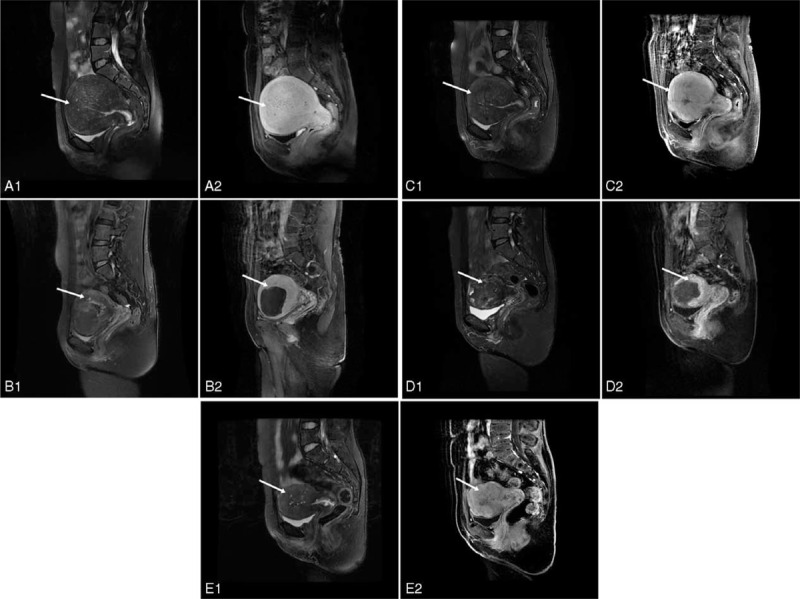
A 44-year-old woman who underwent twice USgHIFU ablation for symptomatic adenomyosis. (A1-A2) Sagittal view of MR image obtained before the first USgHIFU ablation showed diffuse junctional zone thickening of the uterus (arrows). The volume of the uterus was 581 cm^3^. (B1–B2) Sagittal view of MR image obtained 3 months after first USgHIFU ablation showed decreased size of the uterus. The volume of the uterus was 371 cm^3^. Arrows indicate nonperfusion area (183 cm^3^) after USgHIFU. (C1–C2) Sagittal view of MR image obtained 48 months after first USgHIFU ablation (before second USgHIFU ablation) showed enhancement of the treated area (arrows). The volume of the uterus was 319 cm^3^. (D1–D2) Sagittal view of MR image obtained 12 months after second USgHIFU ablation showed decreased size of the uterus. The volume of the uterus was 150 cm^3^. Arrows indicate the nonperfusion area (54 cm^3^) after treatment. (E1–E2) Sagittal view of MR image obtained 24 months after second USgHIFU ablation showed enhancement of the treated area (arrows). The volume of the uterus was 122 cm^3.^ After twice USgHIFU, the patient was asymptomatic until she was menopausal. MR = magnetic resonance, USgHIFU = ultrasound-guided high-intensity focused ultrasound.
